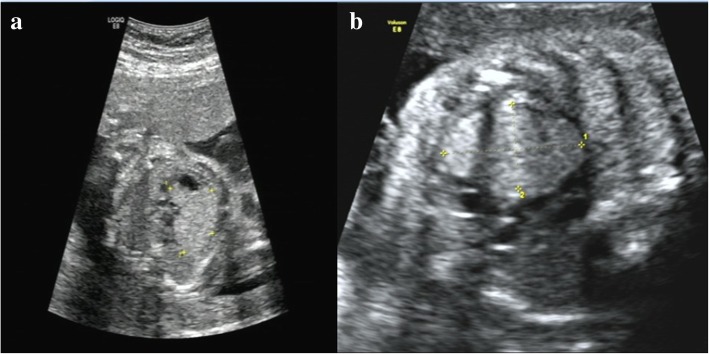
Color Doppler ultrasound imaging. a on November 3, 2017 (23 + 4 weeks of gestation), color Doppler ultrasound imaging showed a hyperechogenic mass in the fetal left ventricle, measuring 1.8 cm × 1.57 cm, broadening of the left lateral ventricle (1.11 cm) and a strong dot-like echo in the left ventricle; b on December 7, 2017, color Doppler ultrasound reexaminations displayed multiple strong echoes in the fetal left ventricle (measuring 3.3 cm × 2.03 cm), compression of the left ventricular outflow tract, obvious enlargement of the tumor, and a 0.92 cm internal diameter of posterior horn of the left lateral ventricle
